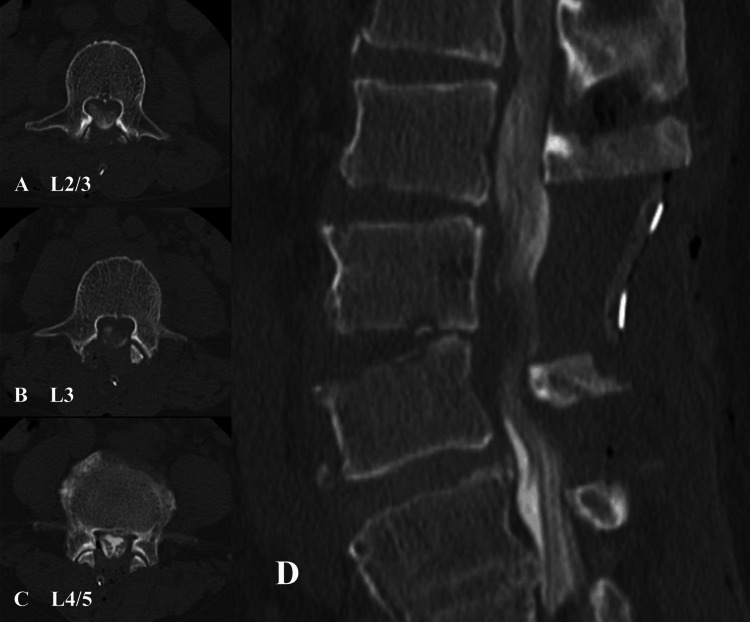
Pyogenic spondylitis. Postoperative CT myelography. Fenestration of L2/3 (A) and L4/5 (C), and laminectomy of L3 (B) were performed. The joint capsule and articular processes of the L3/4 facet joint were destroyed and protruded; they were removed due to necrosis. A purulent substance was evacuated from the dorsal side of the epidural space, and the hypertrophied yellow ligament was resected. After debridement, compression on the dural sac was completely removed (D). The posterior longitudinal ligament was intact, so it was preserved to support spinal stabilization.
